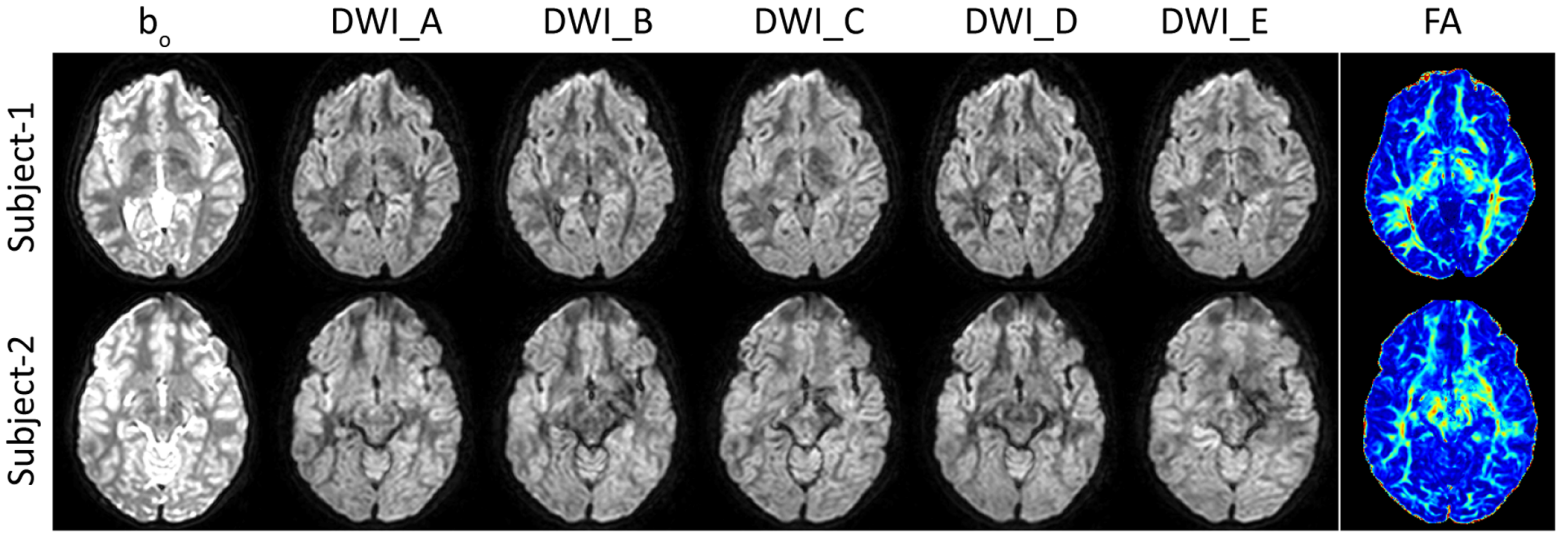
Example input and output data for two subjects from Study II. The DWIs were randomly chosen by a computer but are from the same gradient for each subject. DWIs and b_o_ are shown at different intensity scales for viewing purposes. The FA color scale ranges from [blue-red] and colors map to FA values [0 1]. Inter-subject registration was not performed and the axial slices are from approximate matching locations. The FA map of subject 2 contains an anomalous bright region in the middle-right mid-brain/hemisphere (patient right is image right). This bright region is not clearly traced to DWI artifacts, but upon comparison to a re-scan of the same individual is associated with flow artifacts rather than pathology.
